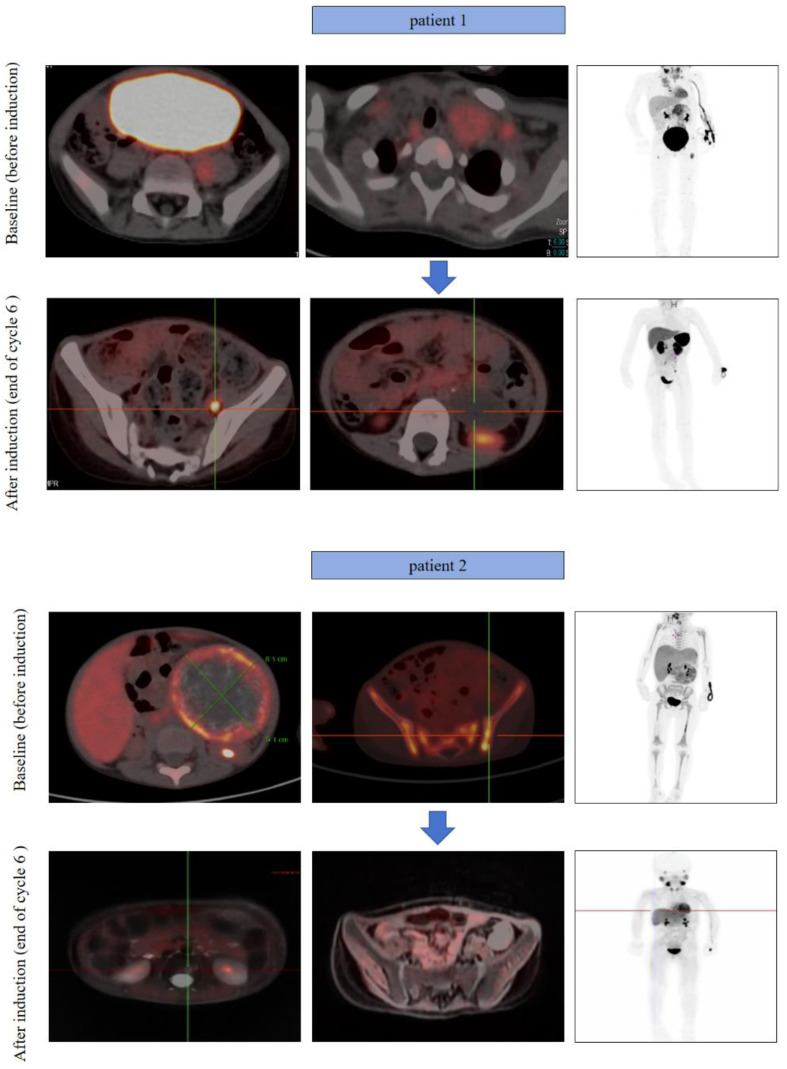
Radiological response before and after induction therapy in two patients with high-risk neuroblastoma. Baseline imaging demonstrated extensive primary tumors and metastatic lesions, while post-induction imaging showed marked regression. Different imaging tracers were used due to clinical practice considerations. Patient 1 underwent baseline imaging with 18F-mFBG and follow-up imaging with DOTA-PET/CT, whereas Patient 2 underwent baseline imaging with 18F-FDG PET/CT and follow-up imaging with 18F-mFBG. Despite these differences, both patients showed consistent and significant reduction in tumor burden.
